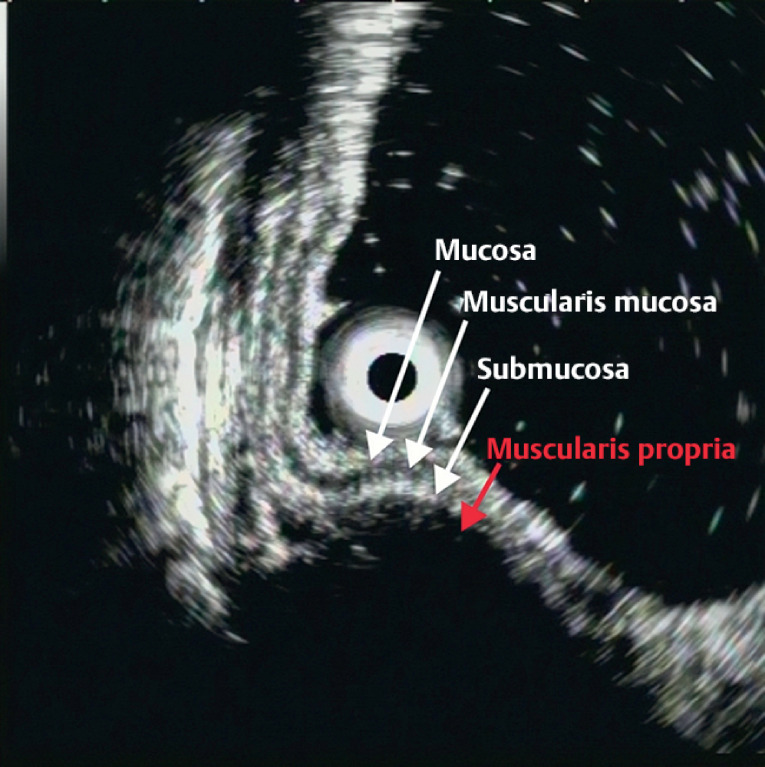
Endoscopic ultrasonography showed a hypoechoic tumor with a clear boundary and an endoluminal growth pattern, which originated from the muscularis propria.
